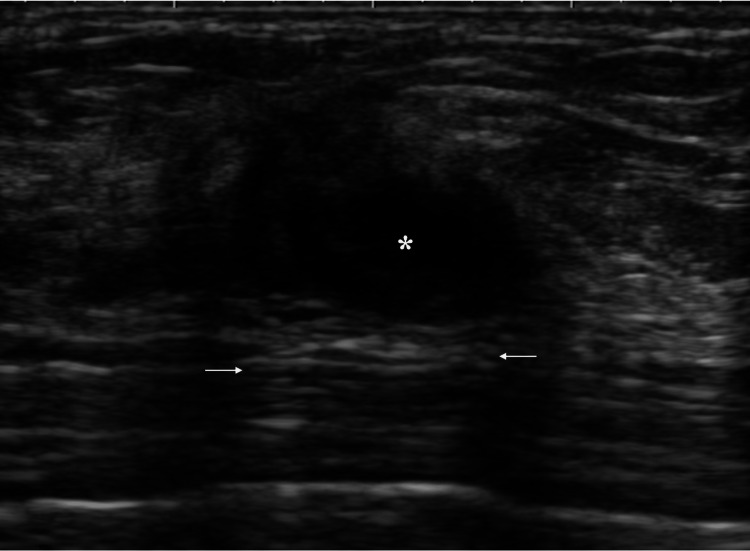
Ultrasound findings Ultrasound showed an oval mass which had partial very low internal echoes (asterisk), enhanced posterior echoes (arrows), and indistinct anterior and lateral tumor borders.
